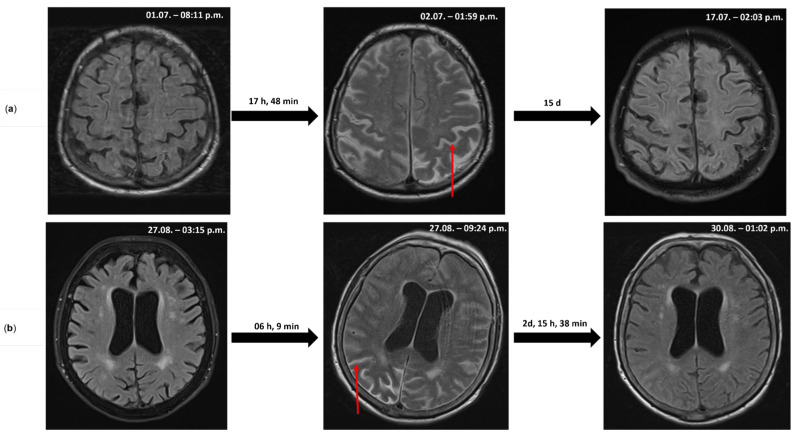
Serial cerebral MRI scans (T2/FLAIR): The initial MRI on admission (left) demonstrates normal CSF signal. Following rapid clinical deterioration, repeat imaging (middle) revealed acute sulcal hyperintensities (example: red arrow), likely representing proteinaceous, inflammatory CSF accumulation secondary to acute blood–brain barrier disruption. Follow-up imaging (right) shows resolution of these signal changes over time. (a) Case 1 and (b) Case 2. All MR images were acquired under standardized institutional protocols. Gadolinium contrast was administered during each MRI session; the displayed FLAIR/T2-weighted images were obtained prior to the subsequent contrast administration within the same examination. The exact date (day/month) and time of MRI sequence acquisition (hh:mm) are indicated in the upper right corner of each image. d, days; h, hours; and min, minutes.
